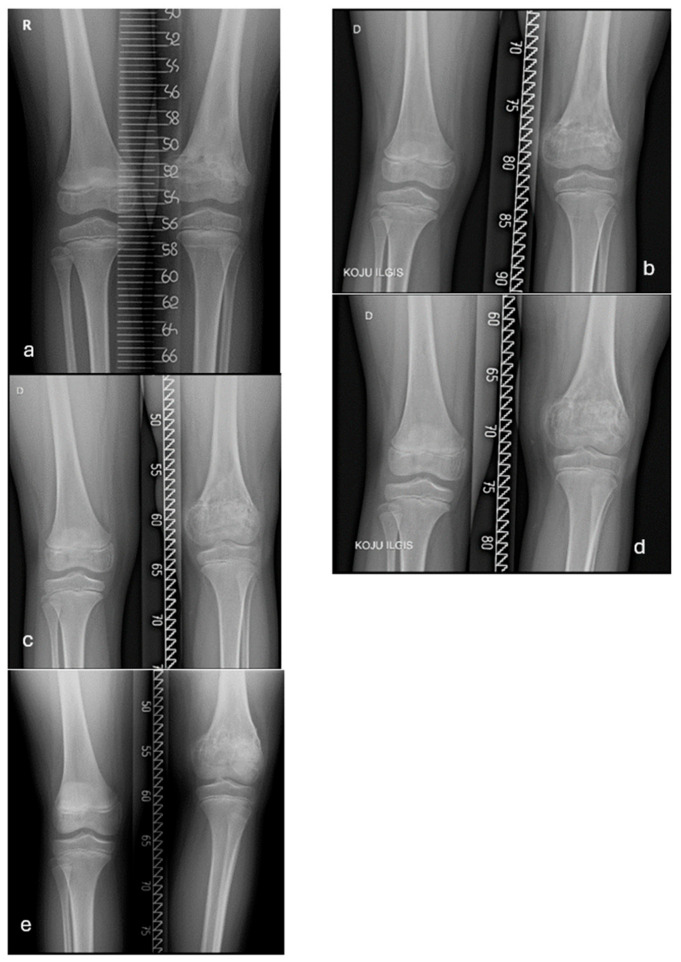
Long-term outpatient follow-up and dynamic assessment of the patient’s growth, anteroposterior radiograph. (a) 10 months post-injury, limb-length discrepancy of 1.3 cm. (b) 18 months post-injury, limb-length discrepancy of 2.3 cm. (c) 36 months post-injury, limb-length discrepancy of 4.1 cm. (d) 3.5 years post-injury, limb-length discrepancy of 2.3 cm. (e) 5 years post-injury, limb-length discrepancy of 6.5 cm.
